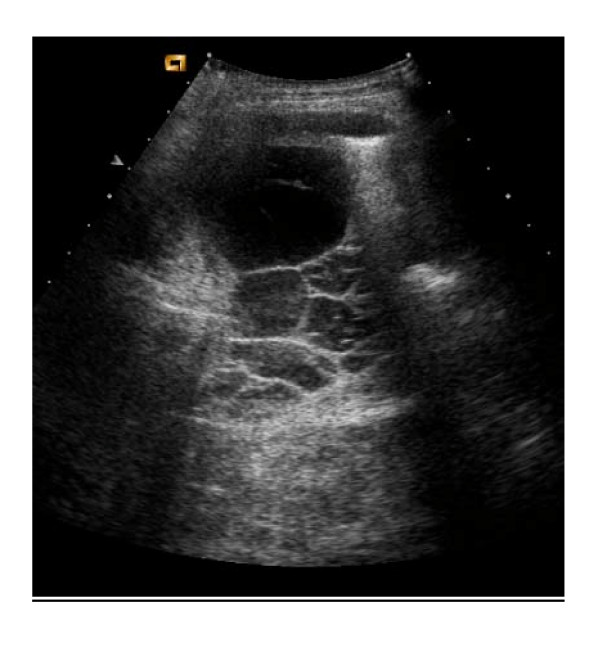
Ultrasound of Thorax, January 2007.
